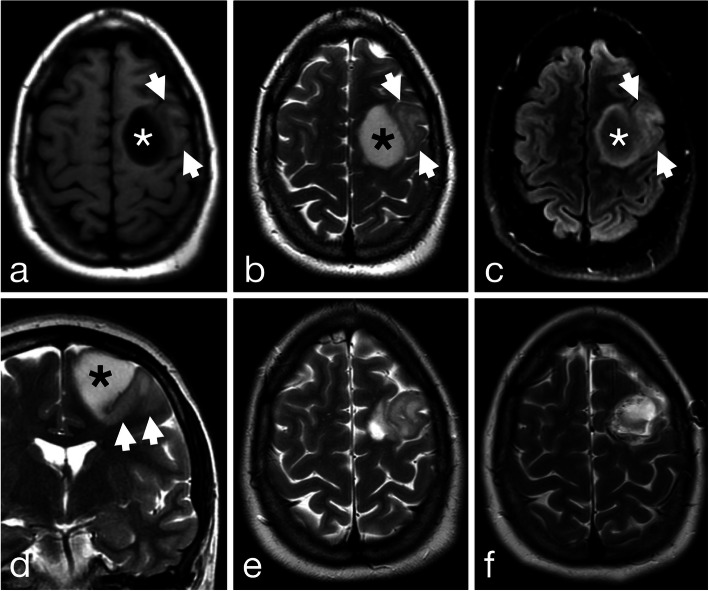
High left frontal tumor with two radiologically distinct components on preoperative axial T1-weighted (a), T2-weighted (b), FLAIR and coronal T2-weighted (d) MR images. A medial T1-hypointense T2-hyperintense component (asterisk) centered in the superior frontal gyrus shows well defined margins and the “T2-FLAIR” mismatch sign indicative of IDH1 mutant 1p/19q non-codeleted astrocytoma. A contiguous more lateral component (arrowheads) centered in the middle frontal gyrus shows less well-defined margins with cortical infiltration and gyral expansion more typical of oligodendroglioma. Follow-up axial T2-weighted imaging after resection of the more medial component (e) and the more lateral component (f)
